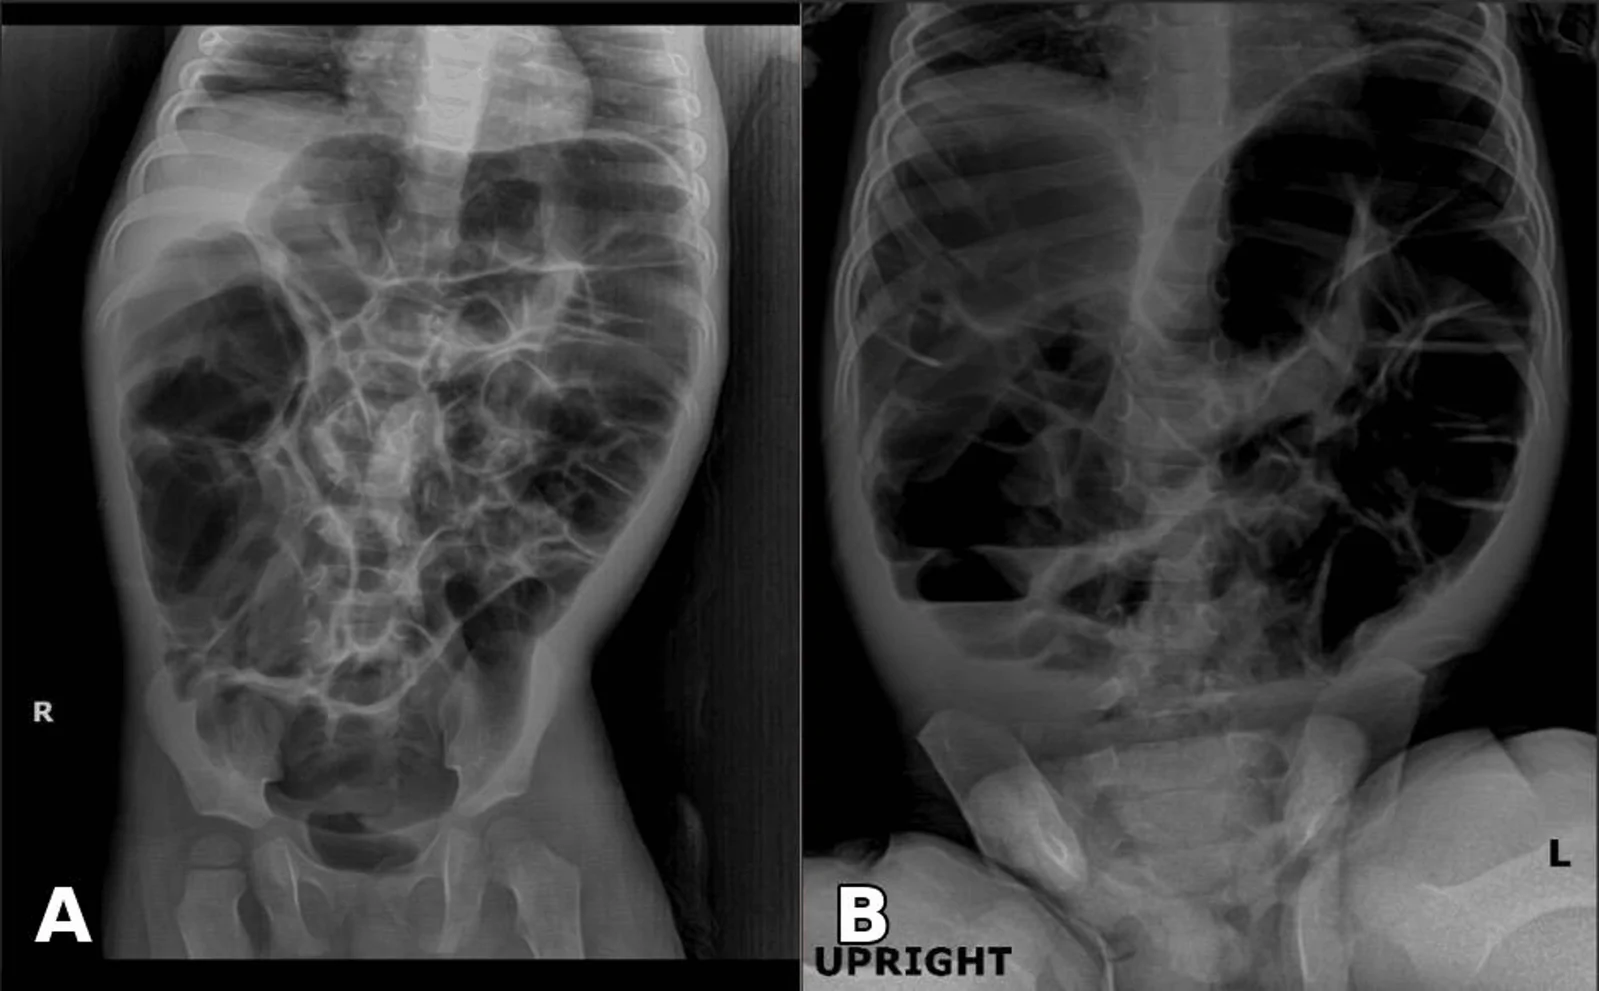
Abdominal radiographs demonstrating diffuse bowel dilation consistent with pseudo-obstruction on index admission. (A) Supine abdominal radiograph demonstrating diffuse gaseous dilation of multiple bowel loops without evidence of pneumoperitoneum or a discrete mechanical obstruction. (B) Upright abdominal radiograph demonstrating multiple dilated bowel loops with air-fluid levels in the absence of a mechanical obstruction.
